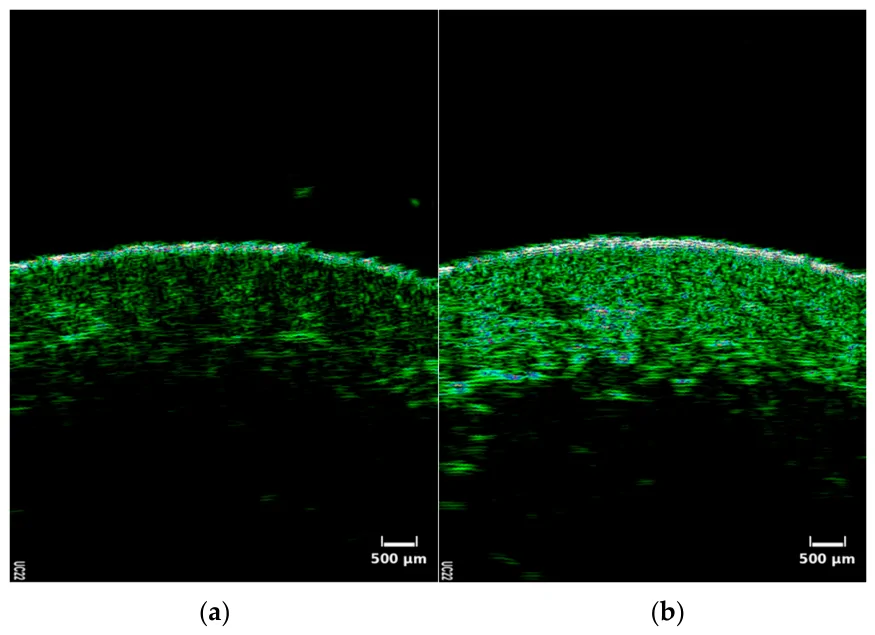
High-frequency ultrasound (22 MHz) images of facial skin at baseline (a) and after 8 weeks of 0.247% retinol treatment (b) in a representative subject, showing increased dermal echogenicity and epidermal definition. Scale bar = 500 µm.
